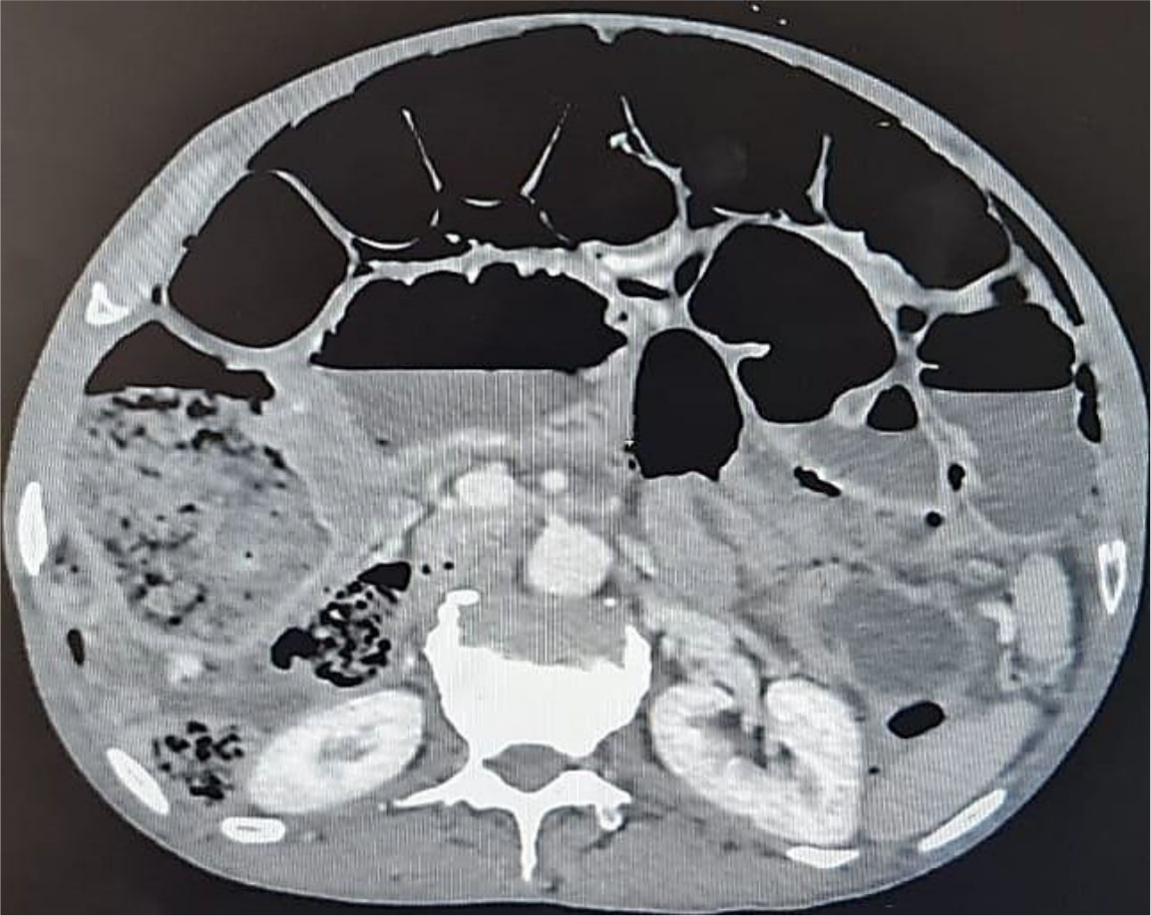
Multiple bubble lesions among ileal loops on Abdominal CT.
